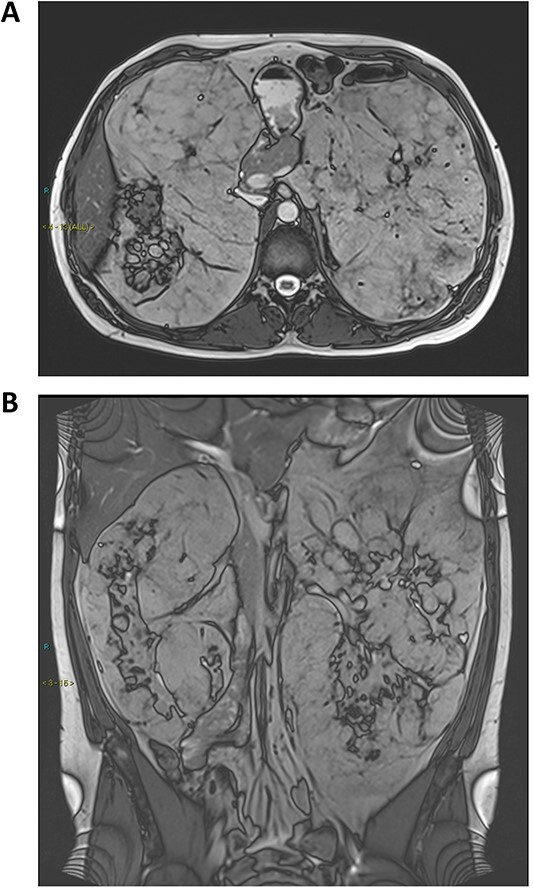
(A) Axial and (B) coronal sections from an abdominal MRI scan showing bilateral massive renal angiomyolipomas and loss of normal kidney anatomy.
